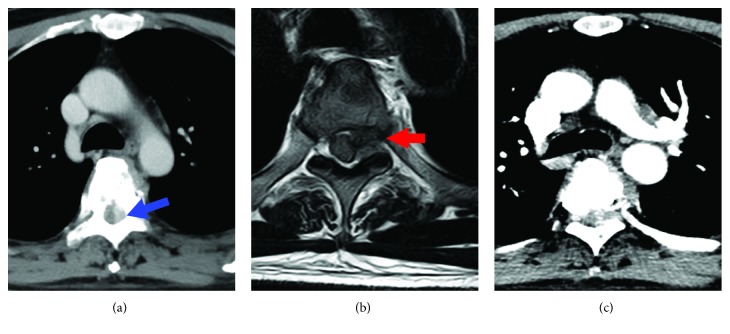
(a) The postoperative CT shows bone cements and metallic implants in T4 without any leakage in the T4 vertebrae body. (b) The postoperative MRI shows a larger intraspinal space-occupying lesion in the T4 intraspinal level. (c) The enhanced CT indicated the possibility of tissue tumor. The blue arrow and red arrow indicate the possibility of tissue tumor or hematoma.
